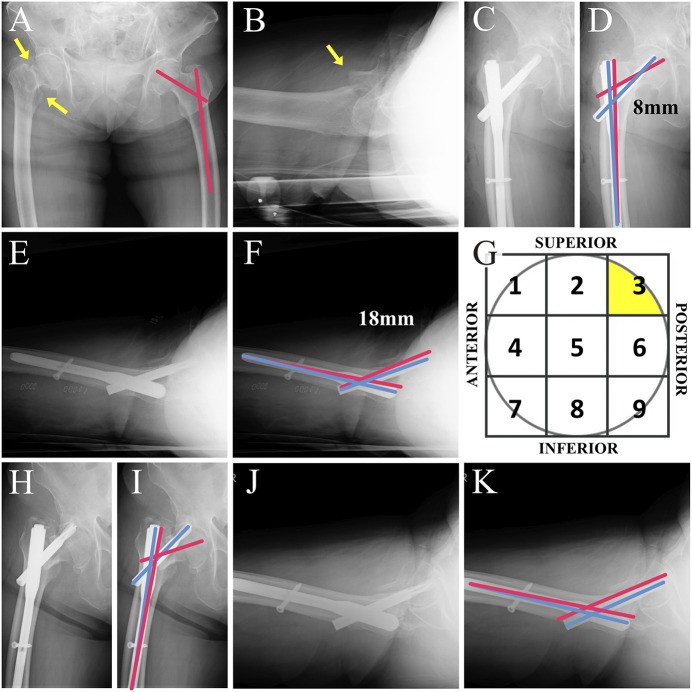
An 80-year-old woman with an intertrochanteric fracture of the right hip who had a cutout at 6 weeks after implantation with a PFNA. (Reproduced, with modification, from the Journal of Typical Medical Images and Videos. Video 2016: Case 178. http://the jtmiv.com. Reproduced with permission.) Anteroposterior (Figs. 3-A, 3-C, 3-D, 3-H, and 3-I) and lateral (Figs. 3-B, 3-E, 3-F, 3-J, and 3-K) radiographs made at the time of admission (Figs. 3-A and 3-B), immediately after PFNA implantation (Figs. 3-C through 3-F), and 6 weeks after the implantation (Figs. 3-H through 3-K). Ideal and practical axes for the lag screw and the nail are indicated with pink and blue lines, respectively. The operation was performed using a 130° nail; however, on retrospective review of this case, we believe a better template would have been a 125° nail. The position of the screw on the femoral head was placed in the superior-posterior zone (zone 3; Fig. 3-G). The TAD was calculated to be 26 mm (Figs. 3-D and 3-F), and the malreduction remained on the lateral radiograph (Figs. 3-J and 3-K). Unfortunately, the patient had a cutout at 6 weeks after the implantation (Figs. 3-H through 3-K).
